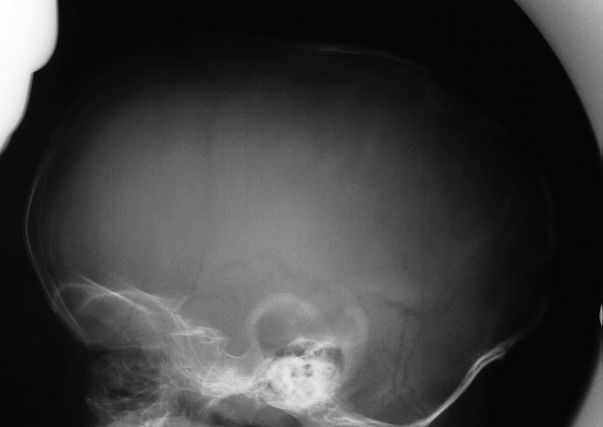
Skull X-ray in scaphocephaly lateral imaging
